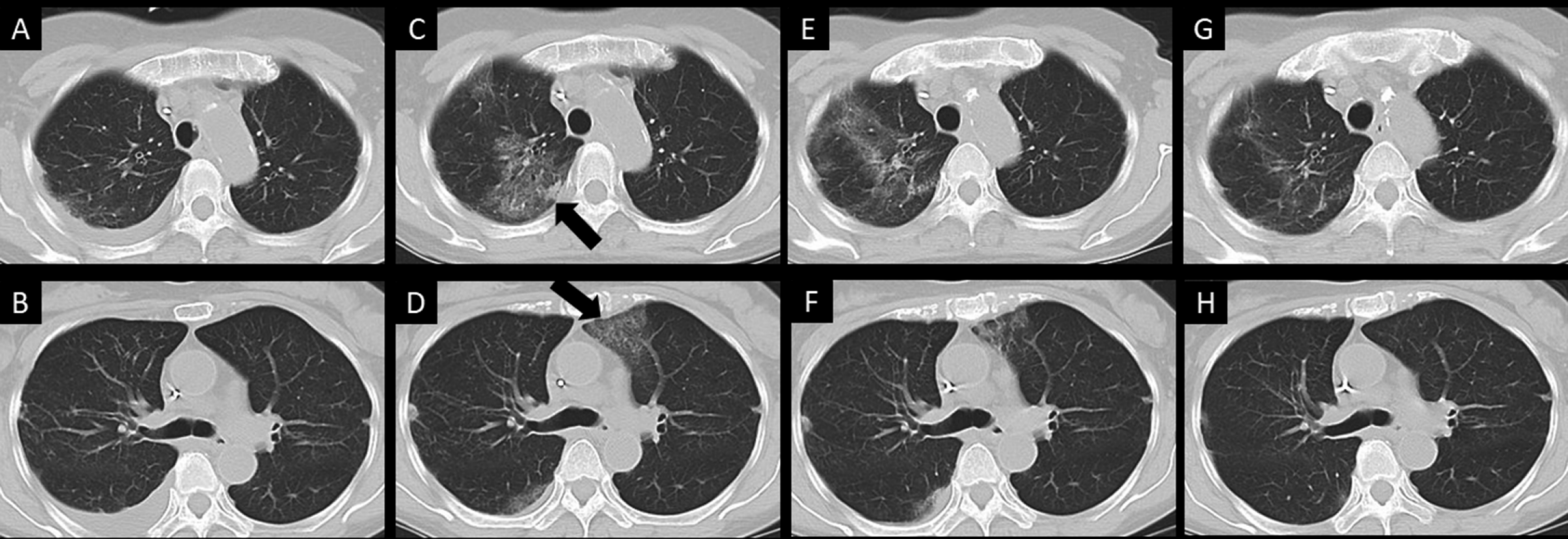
Chest computed tomography (CT). Ground‐glass opacities (GGO) were not observed in panels A and B at day 26 after autologous peripheral blood stem cell transplantation. After nine days, when the patient exhibited respiratory symptoms, GGO was detected in the bilateral lung field (panels C and D). In the CT performed on the seventh day after starting the treatment, the GGO abated. Furthermore, the GGO completely disappeared in the CT performed on the 18th day after starting the treatment.
